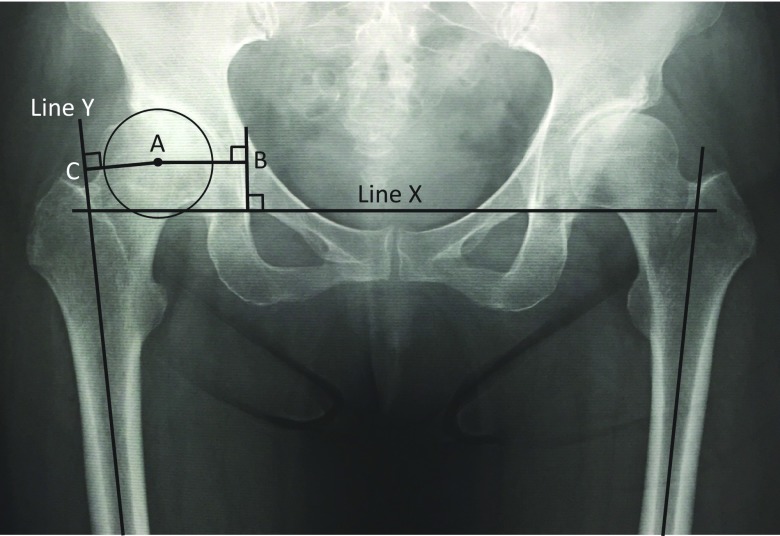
An anteroposterior radiograph of the hips was obtained in a supine position immediately preoperatively. X: trans-teardrop line; Y: central axis of the femur; Z: central axis of the femoral neck; point A: center of the femoral head; AB: acetabular offset; AC: femoral offset. The total offset is the sum of lines AB and AC [10].
